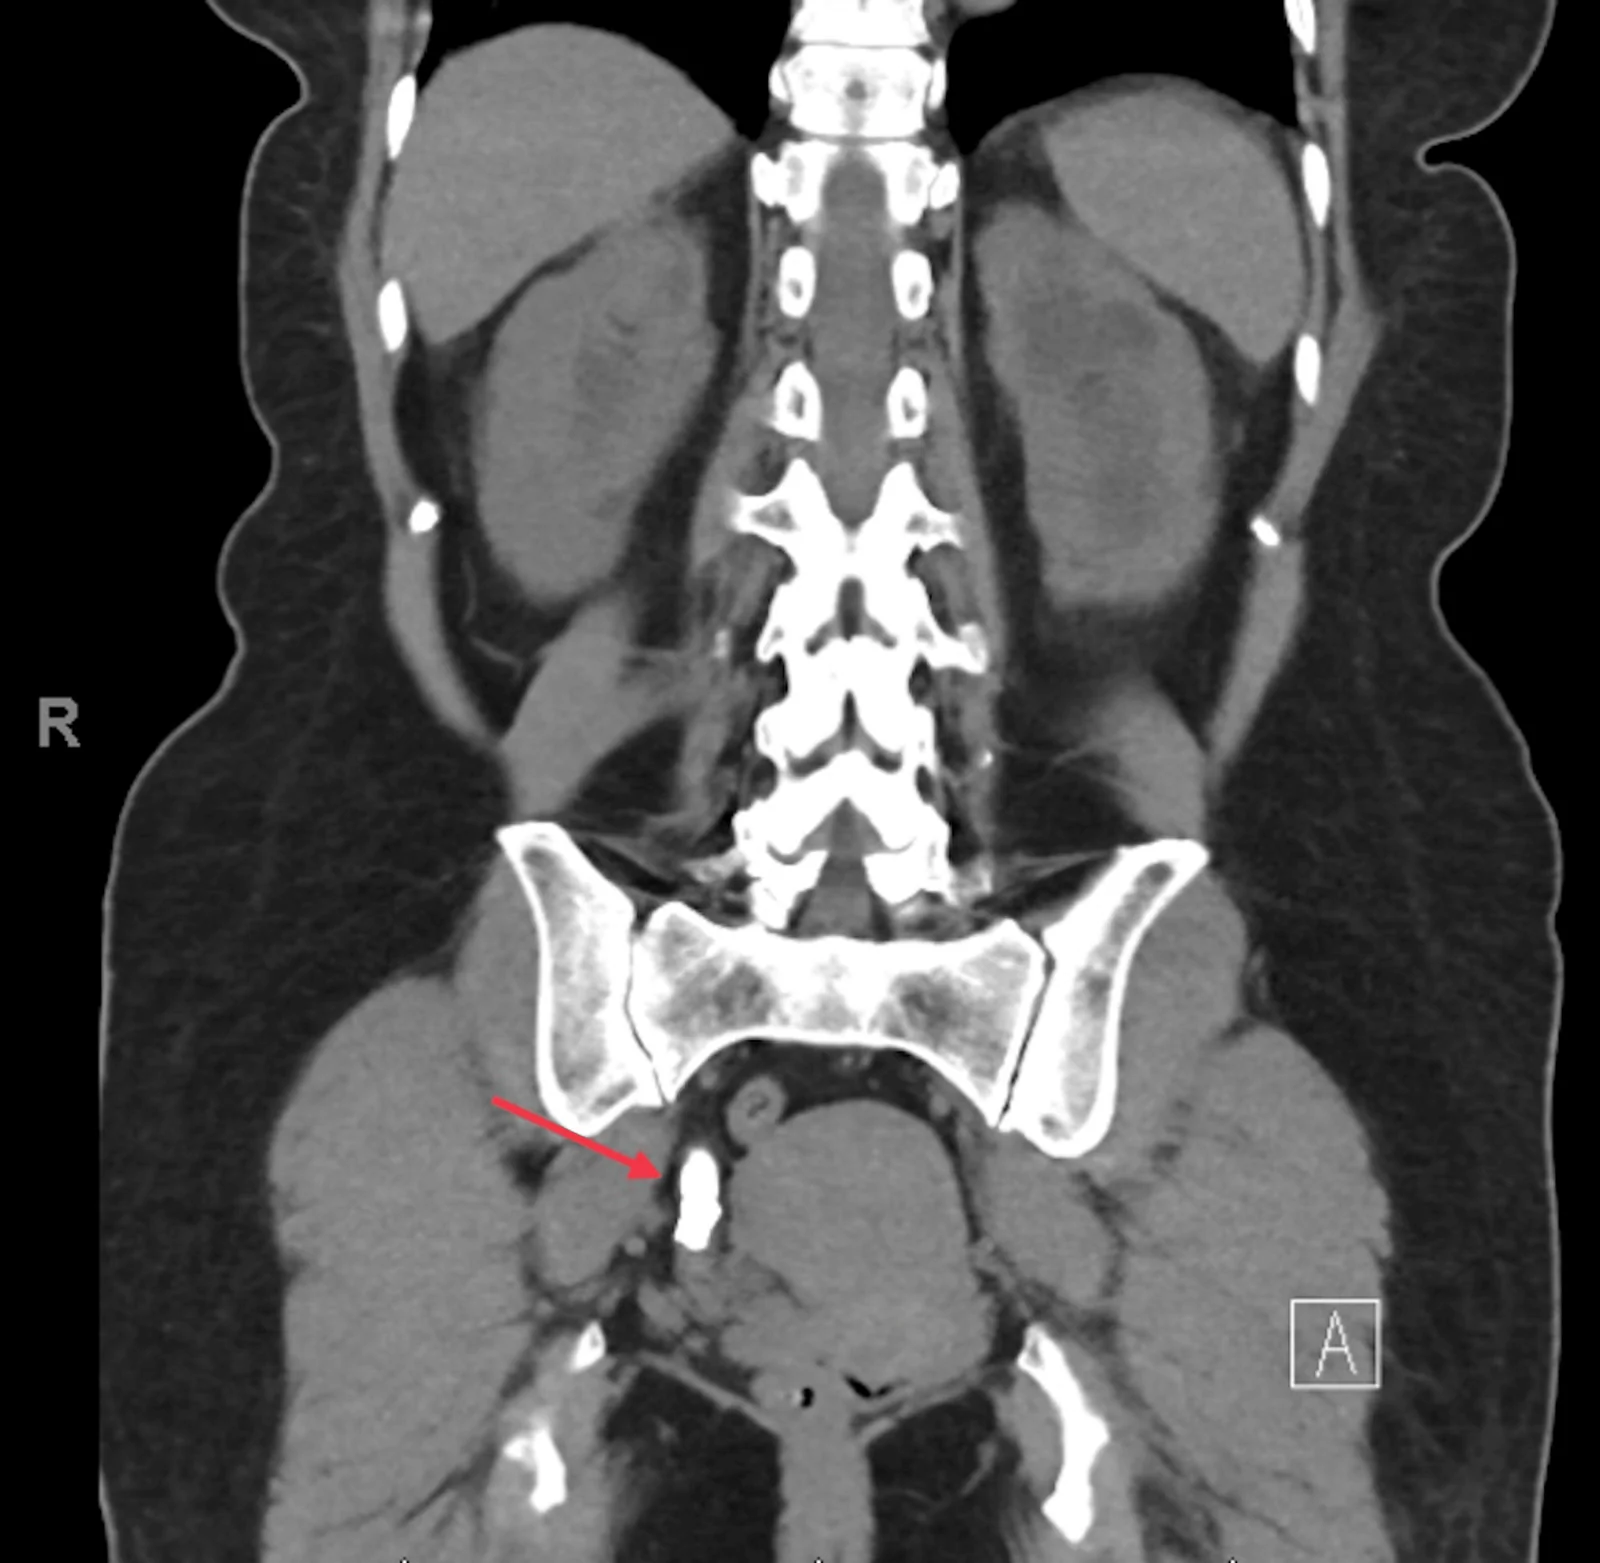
Coronal non-contrast CT image showing right distal ureteral stones. The red arrow indicates one of the stones.
